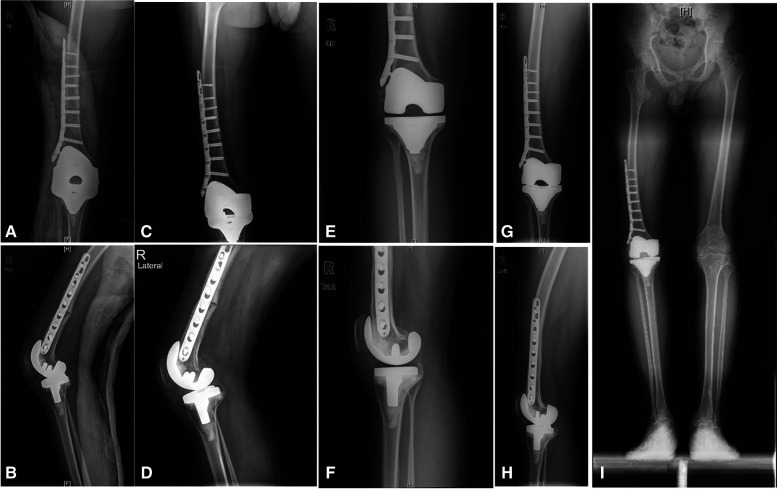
(A,B) Postoperative knee and femur x-rays at 3 days; (C,D) postoperative x-rays at 7 months; (E–H) postoperative x-rays at 23 months; (I) full-length x-rays of both lower limbs in weight-bearing positions at 23 months after surgery.
